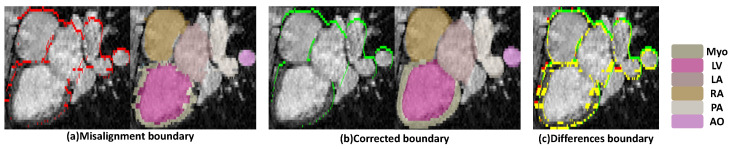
Impact of respiratory motion on MRI, causing inter-slice misalignment and structural deformation. (a) Shows the misaligned anatomical structures, (b) displays the corrected, anatomically accurate structures, and (c) highlights the differences between them, with boundary colors: red for misaligned boundaries, green for correct boundaries, and yellow for differences.
